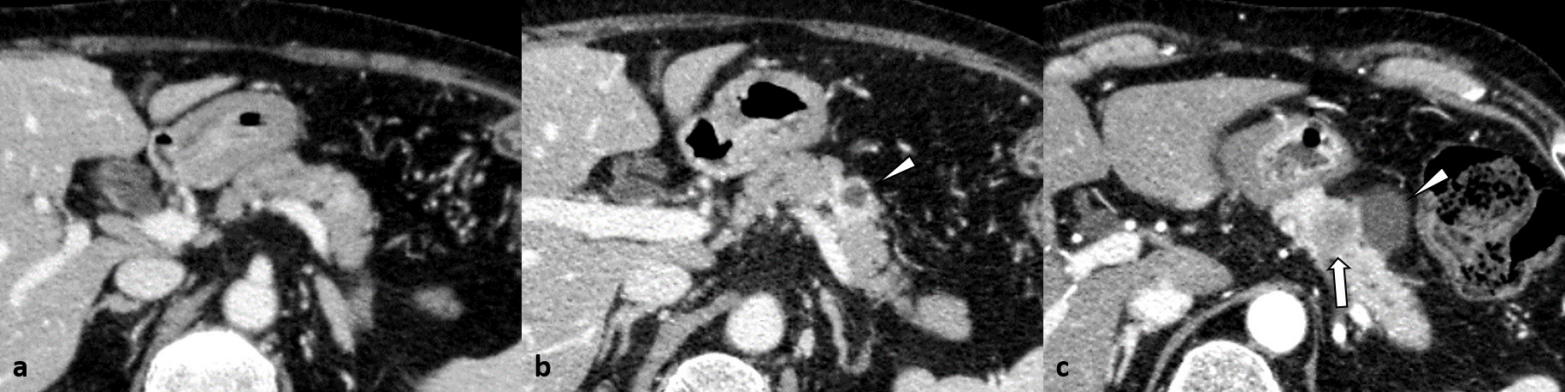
A 61-year-old female with pancreatic adenocarcinoma that developed after treatment for colon cancer (Case 6) (a) Venous phase contrast-enhanced CT image obtained 11 months before the pancreatic surgery showing no abnormal findings suggestive of pancreatic adenocarcinoma. (b) Contrast-enhanced CT image obtained 6 months before the pancreatic surgery showing a pancreatic cyst (11 mm diameter, arrowhead) with surrounding parenchymal enhancement in the pancreatic tail. (c) On triple-phase contrast-enhanced pancreatic phase CT image obtained immediately before pancreatic surgery showing that the pancreatic cyst (arrowhead) has increased in size. There is an obvious ill-defined hypoattenuated pancreatic tail mass (33 mm diameter, arrow) close to the pancreatic cyst. The solid pancreatic mass is invading the peripancreatic fat tissue.
